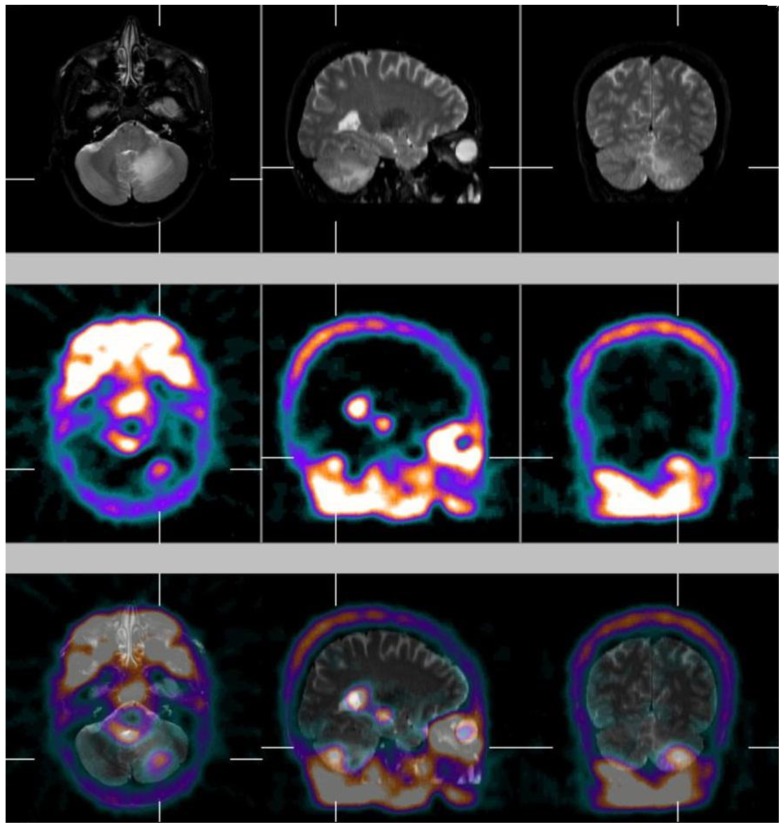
Magnetic resonance imaging (MRI) (at the top), ^99^
